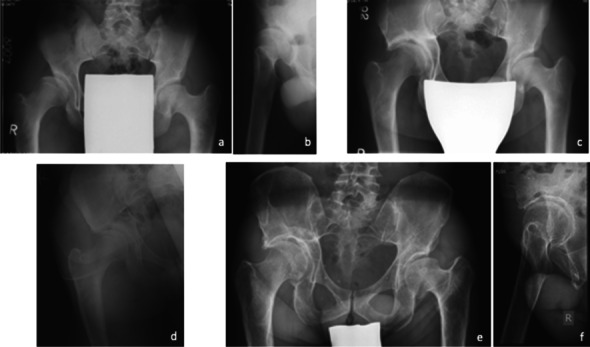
Male patient, CPO at age of 17 years with severe hip dysplasia. The right hip shows a L-CEA of 0 and a A-CEA angle of 14°, OA grade 0 (a, b). After CPO the acetabulum was medicalized by 47.6% and full coverage of the femoral head was achieved (c). The three year post op X-ray shows remodeling of the acetabulum and a congruent joint (d). After thirty years the patient had full ROM, negative Trendelenburg sign and pain of 2.2 out of 10 on the VAS. The L-CEA was 54 and the A-CEA 64°, OA grade 2 (e, f)
